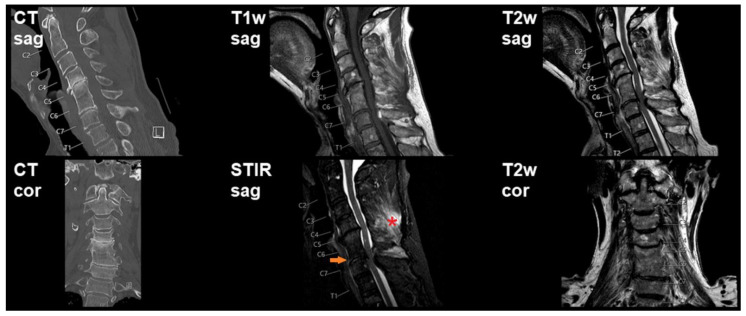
Preoperative imaging shows degenerative changes particularly for the segments C4/C5 and C5/C6 according to computed tomography (CT). Cervical magnetic resonance imaging (MRI), using T1-weighted, T2-weighted, and short tau inversion recovery (STIR) sequences, depicts a small prevertebral hematoma (*orange arrow* in STIR imaging) as well as ligamentous injury and edema in the nuchal soft tissue (*red asterisk* in STIR imaging). Furthermore, spinal stenosis is present at level C6/C7.
